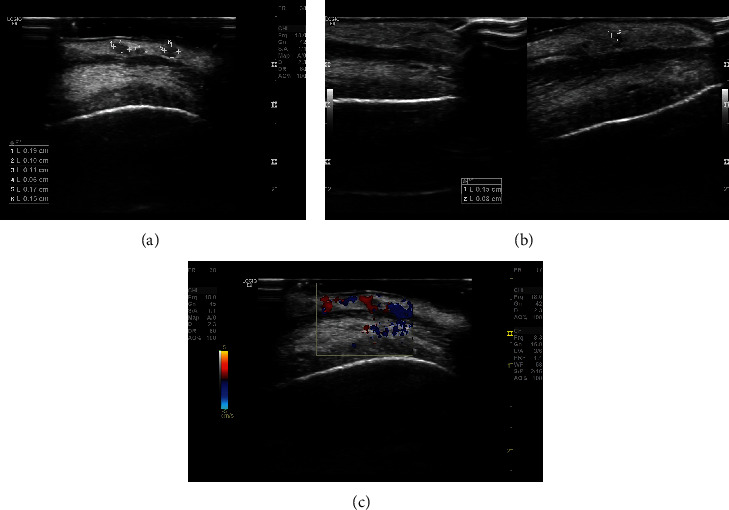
(a, b) Adjacent tiny lesions on the right and left sides of the lip. (c) The color Doppler was suggestive of an avascular anechoic lesion.
